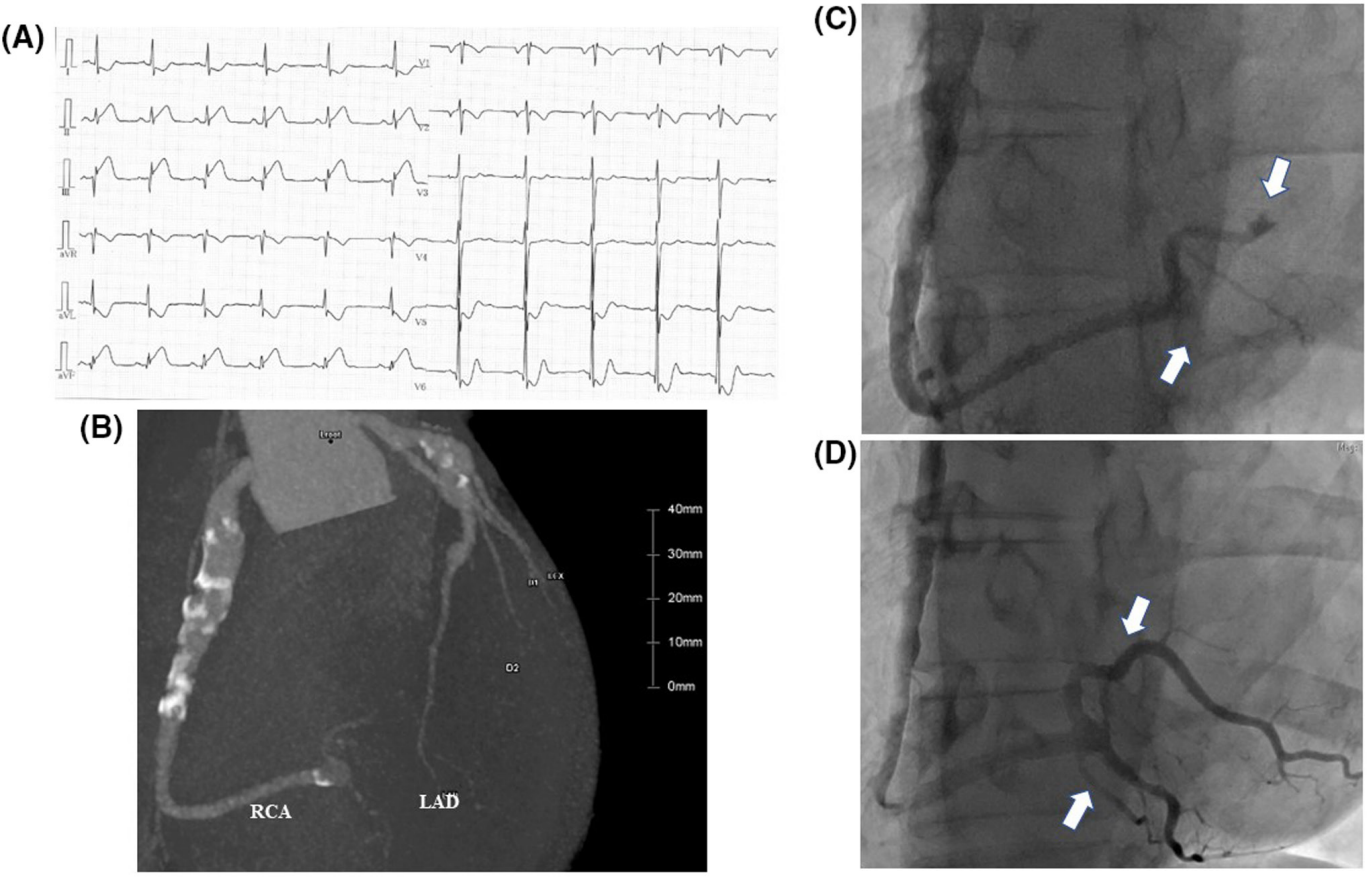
(A) Electrogram shows ST elevation in leads II, III, and aVF. (B) Coronary CT angiography at a regular medical checkup. (C) Coronary angiography revealed a thrombus occlusion in the distal right coronary artery (White arrows). (D) Coronary angiography revealed the disappearance of the thrombus in the distal right coronary artery (White arrows).
